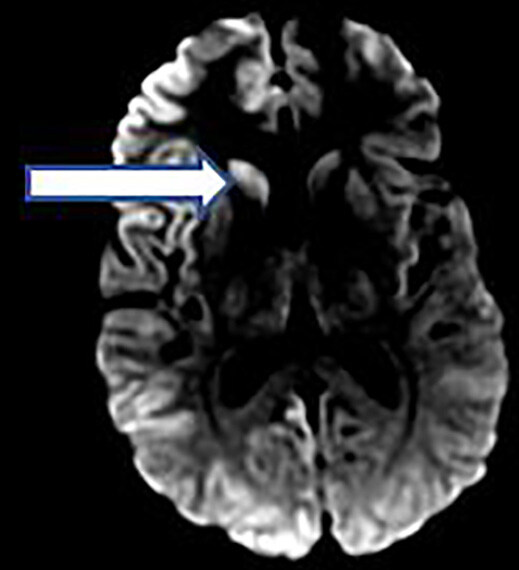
A single, magnetic resonance imaging slice of diffusion-weighted imaging of the patient’s brain. The image shows high-intensity signaling in the right basal ganglia, indicated by the arrow. The high-intensity signaling in the right basal ganglia is consistent with Creutzfeldt-Jacob disease.
